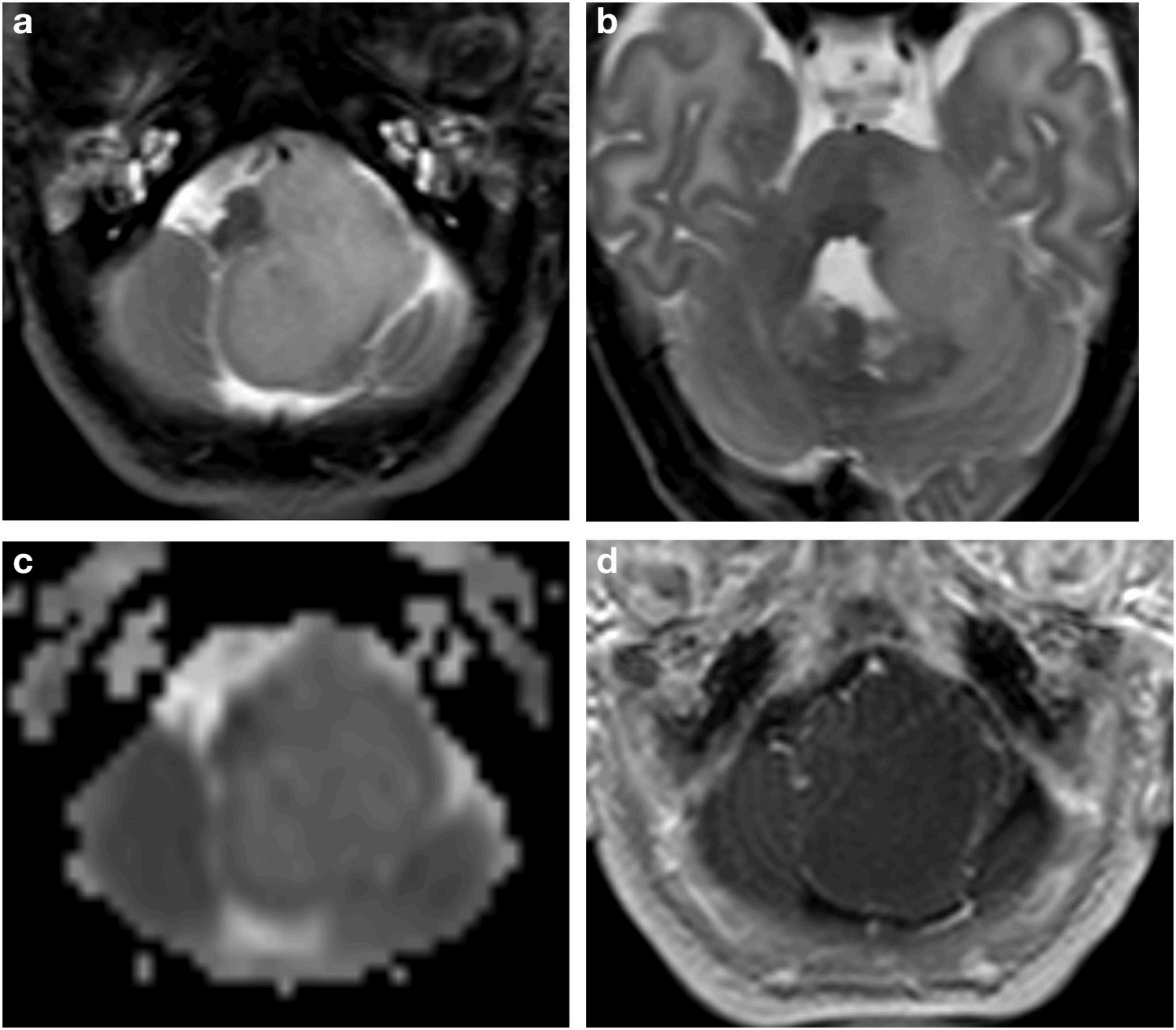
Brain MRI. Axial T2w (a,b) ADC map (c) and Gd T1w (d) images. Extensive hyperintense mass in the left cerebellopontine angle extended upward into the cerebellum with lower peripheral ADC values and subtle peripheral linear irregular enhancement after gadolinium injection. The fourth ventricle, medulla oblongata and the cerebellum are dislocated and compressed.
